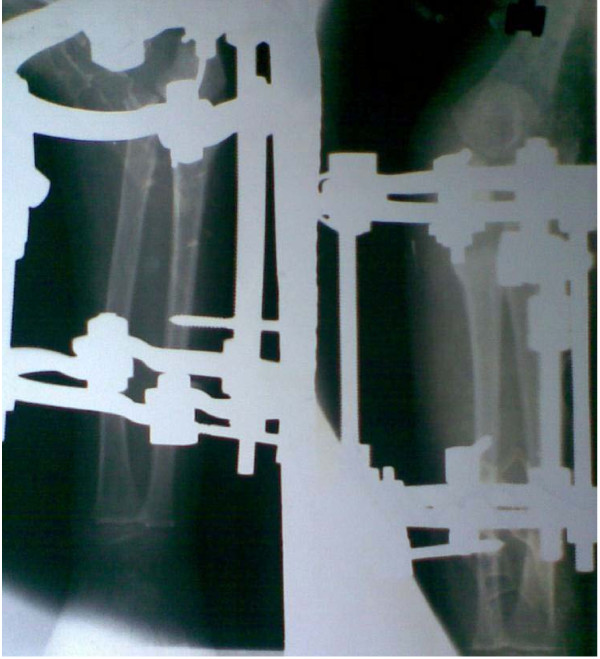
Postoperative radiograph after application of Ilizarov fixator and ulnar osteotomy (Patient 1).
